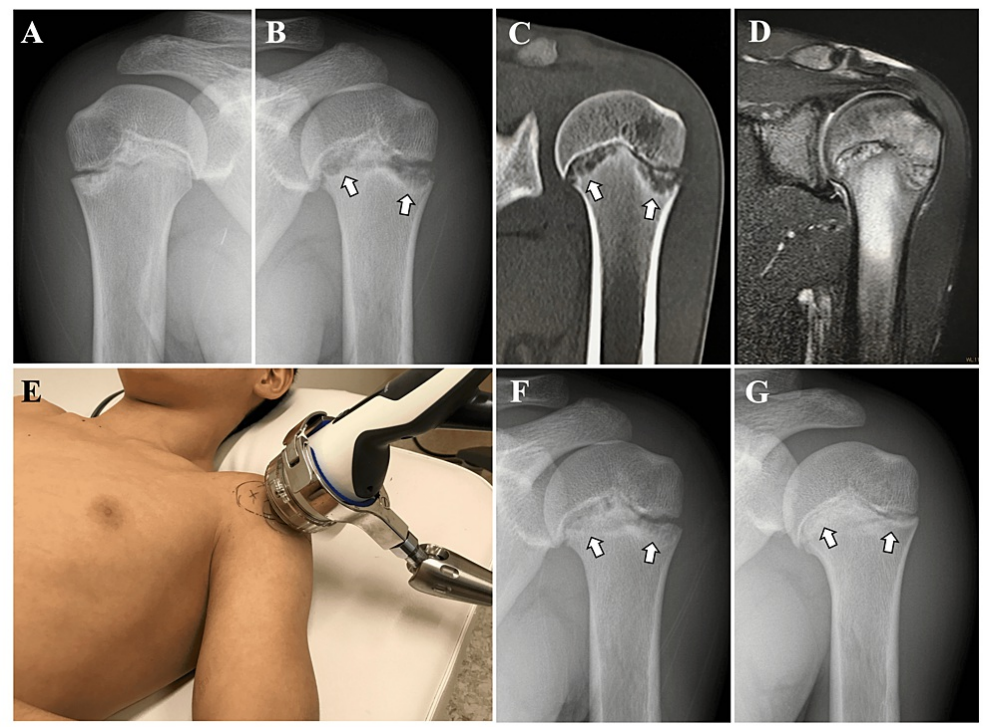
Case 1 A: Radiograph of the healthy side. B, C: Radiograph and computed tomography showed bone resorption on the distal side of the proximal humeral physis (arrows). D: T2-weighted fat-suppressed magnetic resonance imaging showed high signal changes centered around the epiphysis. E: Focused extracorporeal shock wave therapy (ESWT) was applied to the lateral side of the proximal humerus. F: Two months after initiating ESWT, vigorous bone repair was observed (arrows). G: The radiograph seven months after the start of ESWT showed good bone repair (arrows).
